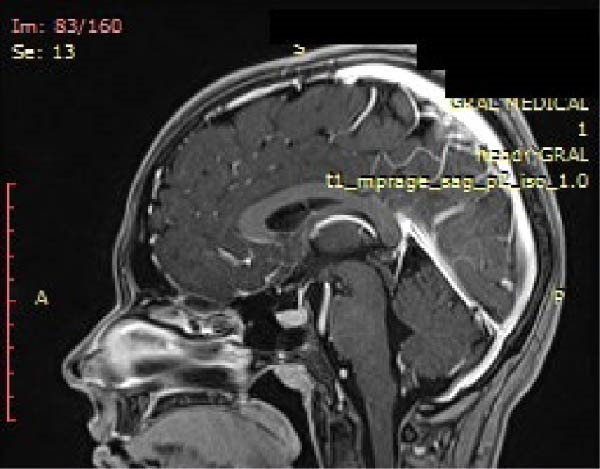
Sagittal T1‐weighted MPRAGE MRI showing a small hypointense lesion within the anterior pituitary gland, consistent with a microprolactinoma. The lesion appears as a subtle and well‐demarcated area disrupting the normal pituitary contour.
